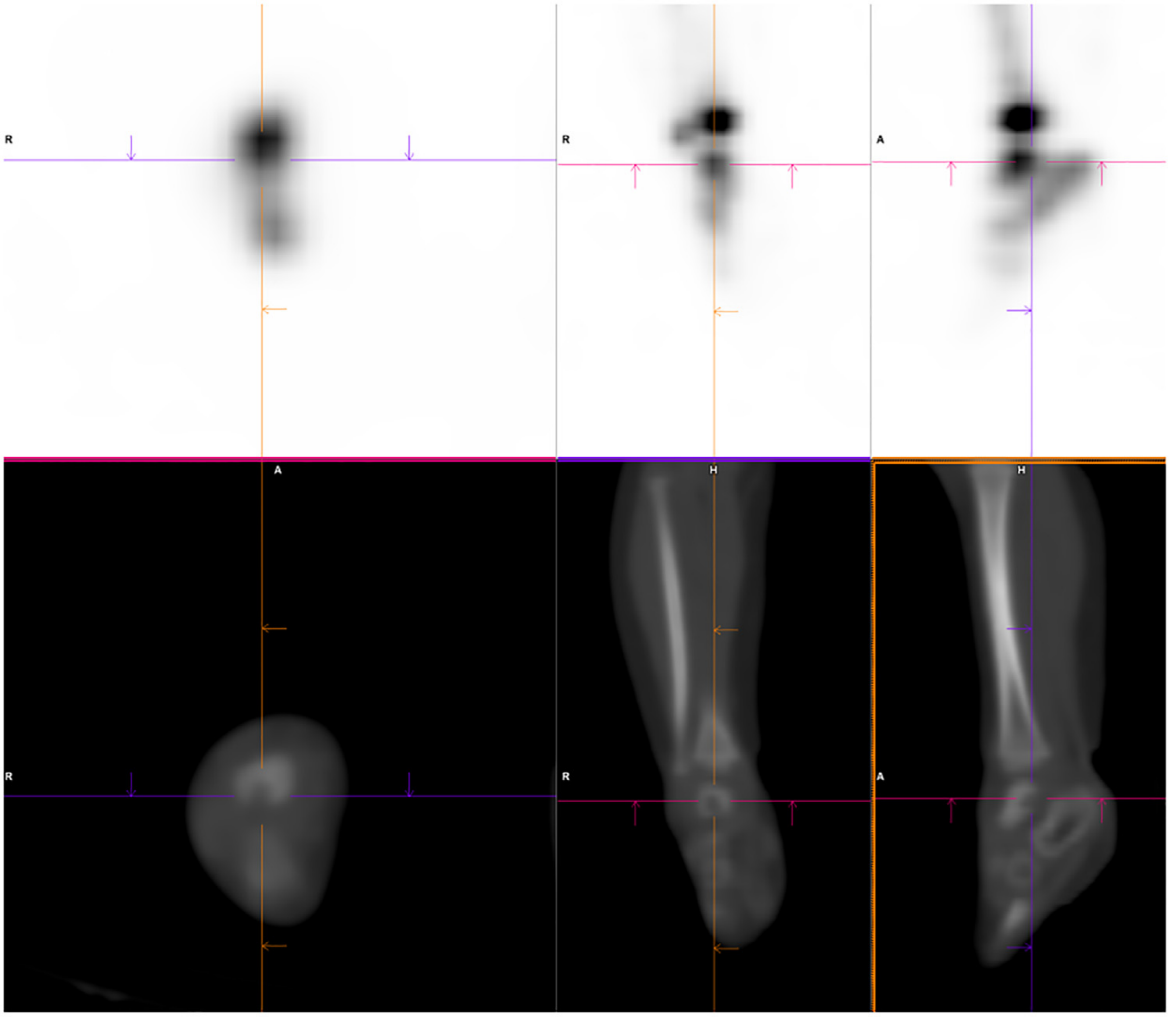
SPECT/CT imaging of the feet (axial, sagittal and coronal views), showing focal increased radiotracer uptake at the site of a well-defined lucent lesion in the posterior aspect of the talus of the right foot.
